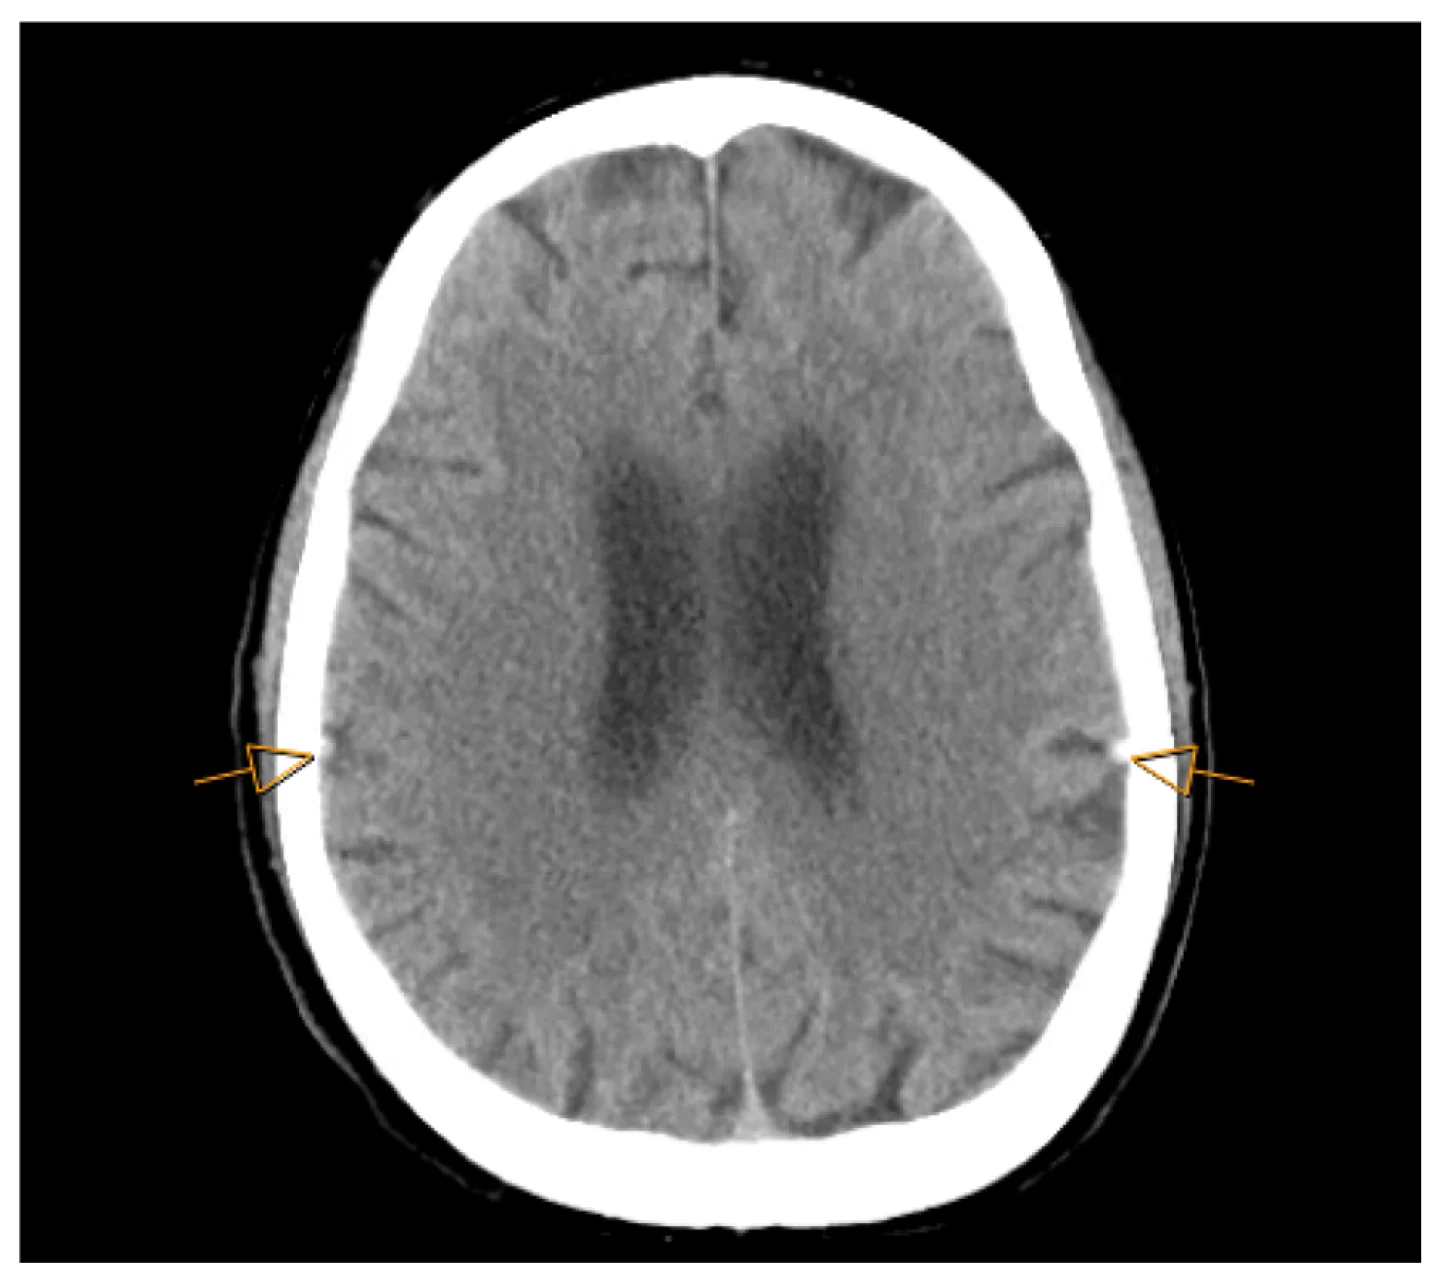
Axial noncontrast CT head demonstrating bilateral hyperdense cortical veins along the parietal convexities (arrows), more pronounced on the left.
